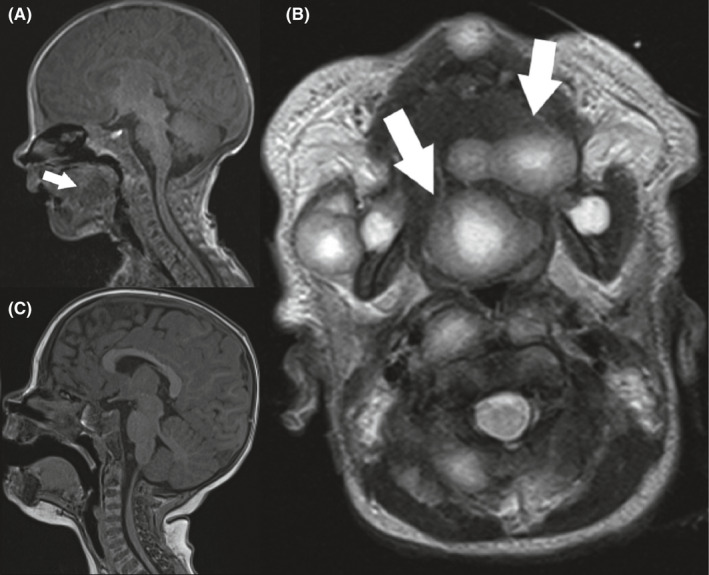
Patient 1 at 2 weeks old demonstrating obstructive, tongue/base of tongue lesion on sagittal T1 MRI (A) and axial T2 (B); arrows designate tongue and base of tongue myofibromas. Sagittal T1 MRI 6 months after cessation of imatinib demonstrating absence of myofibroma (C)
